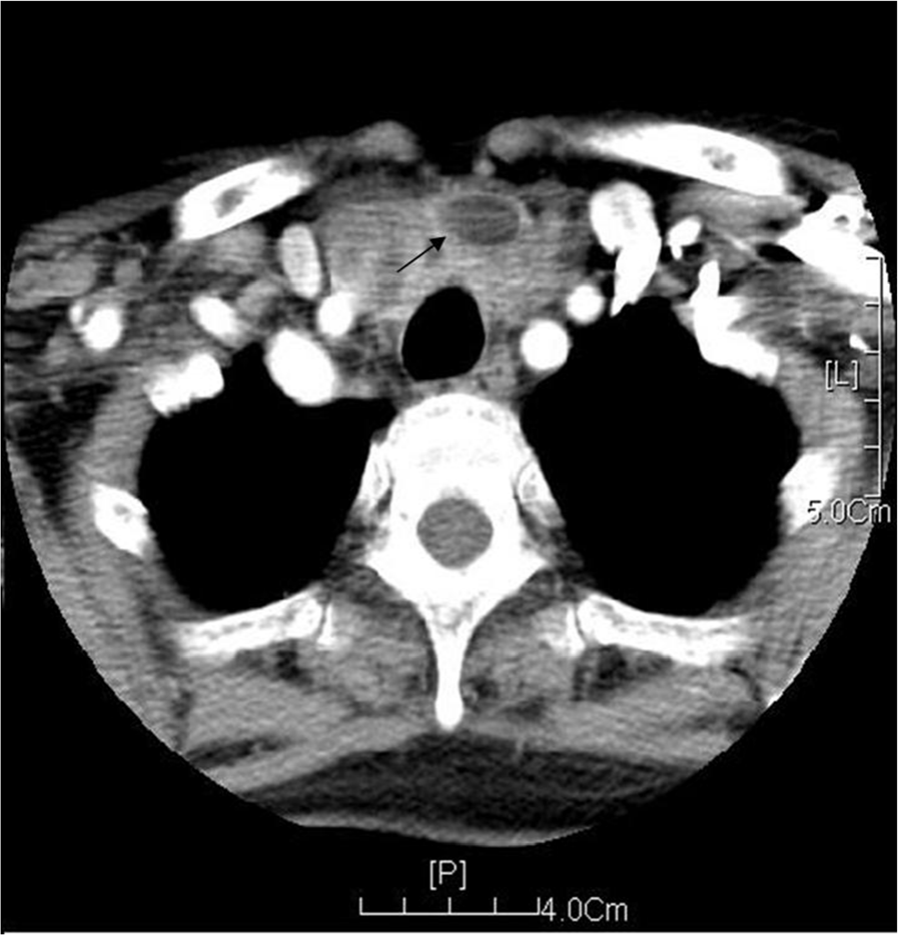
Enhanced CT scanning of the thyroid gland shows a cystic-solid low density mass, which is located underneath lateral lobes of the thyroid gland and manifested as inhomogeneous enhancement (Black arrow). Inside of the mass, a lamellar non-enhanced area is visible. The mass is projected into the gaps of surrounding vessels. The cervical part shows no obvious enlarged lymph nodes.
